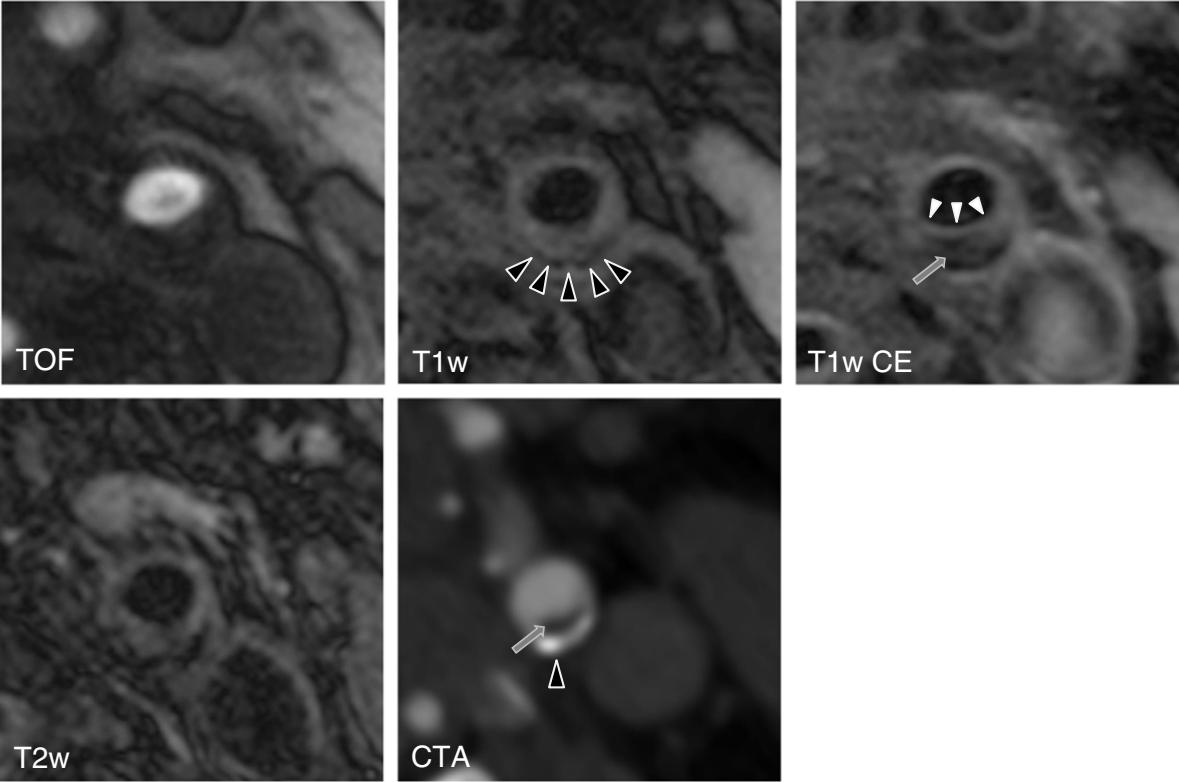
Shows axial TOF, T1 weighted pre- and post- contrast and T2 weighted high-resolution black-blood CMR and CTA (lower right) images of a stable carotid plaque on the asymptomatic left side in a 66 year old patient who had suffered from right hemispheric stroke. Both CMR and CTA images show the presence of an AHA lesion type 7 plaque in the dorsal wall of the left proximal internal carotid artery. After administration of contrast material the thick fibrous cap is delineated as a hyperintense rim in the T1 weighted contrast enhanced images, separating the plaque from the lumen (white arrowheads). Also note the hypointense signal of the plaque interior in the T1 weighted contrast enhanced image as well as the hypodense area in the CTA image corresponding to a large lipid-rich necrotic core (arrow), measured at 166 HU, probably due to blooming artifacts caused by its calcified portion. The hypointense rim in the peripheral plaque in all MR sequences and the corresponding hyperdense area in the CTA image indicate the presence of a calcification (black arrowheads).
